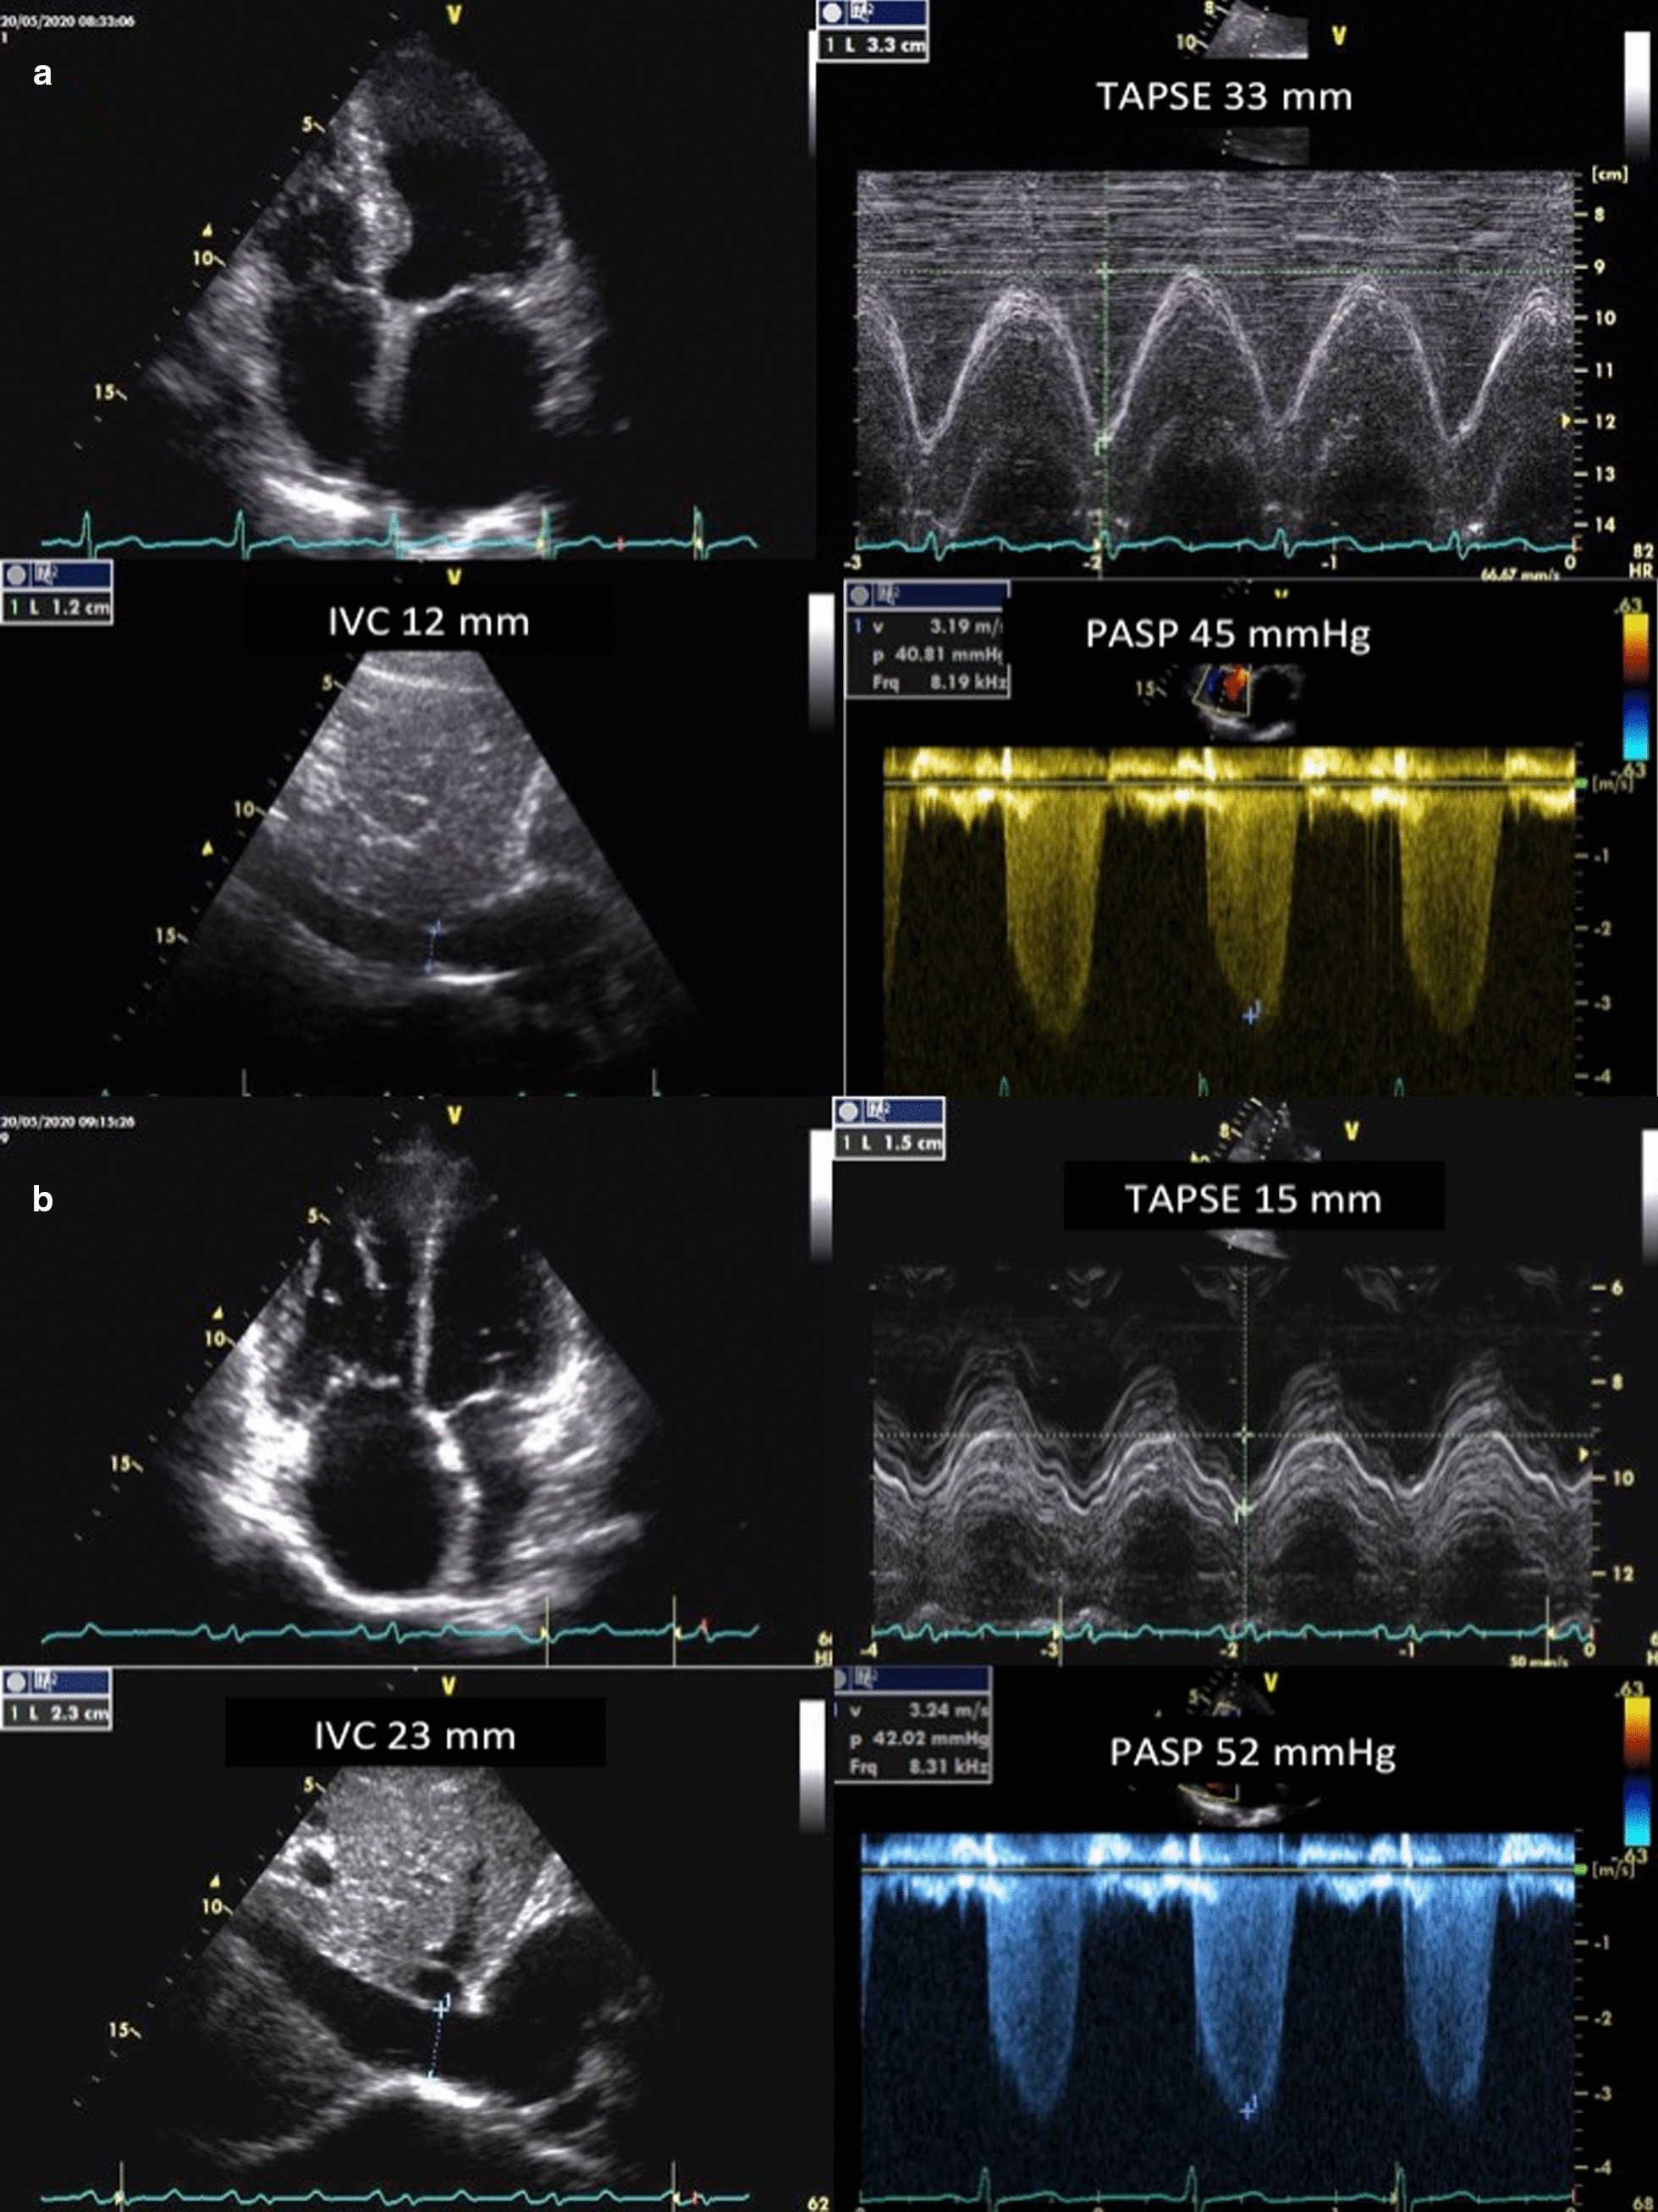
Coupling of right ventricular function to the pulmonary circulation evaluated by the tricuspid annular plane systolic excursion (TAPSE) to systolic pulmonary artery pressure (PASP) ratio. a Normal echocardiographic phenotype with increased pulmonary artery systolic pressure (PASP), normal tricuspid annulus plane systolic excursion (TAPSE), and preserved TAPSE/PASP. b Typical right heart echocardiographic phenotype with increased PASP, reduced TAPSE, low TAPSE/PASP, and right/left ventricular basal diameter ratio > 1. *IVC* inferior vena cava
